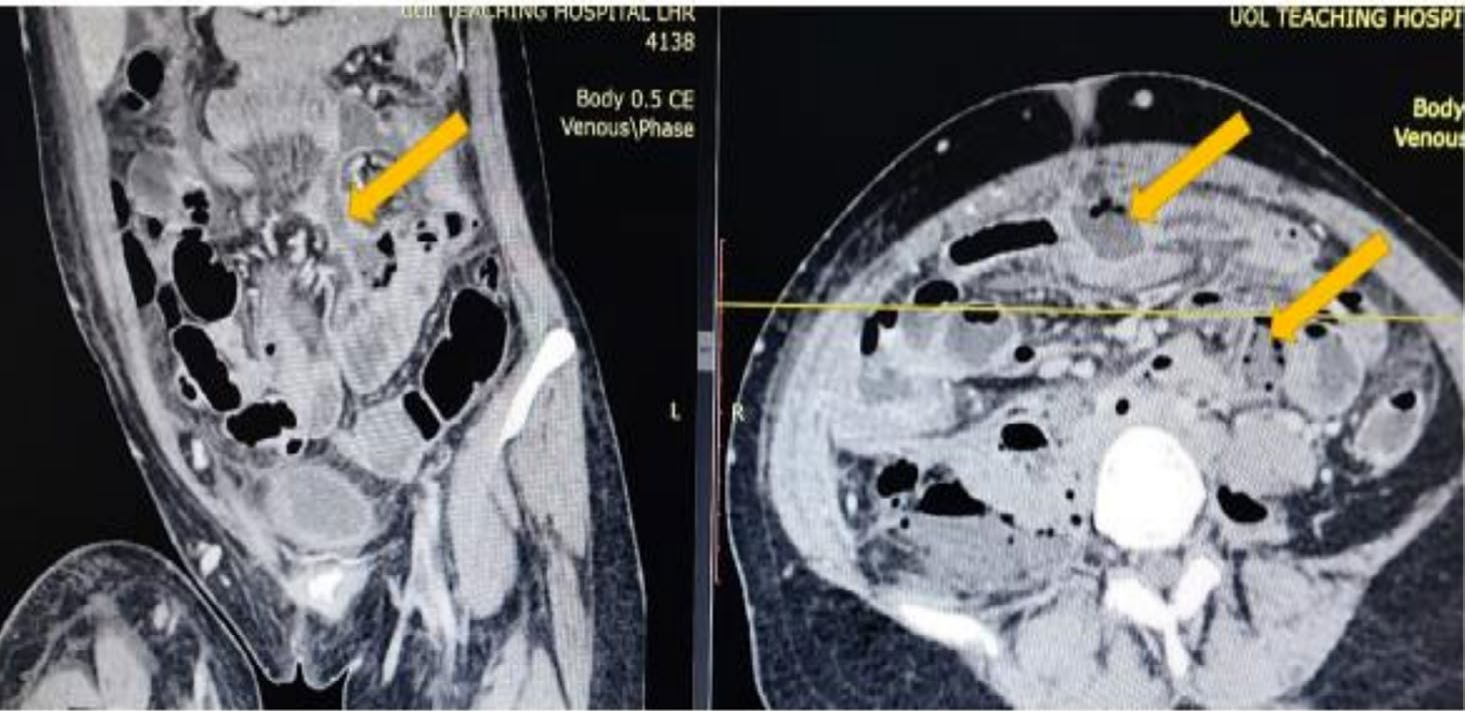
Communication between ileum and abscess cavity. An axial contrast‐enhanced CT image with oral contrast media. The image demonstrates a thickened and inflamed loop of the distal ileum immediately adjacent to the large right‐sided psoas abscess. A faint tract and inflammatory stranding can be seen between the bowel loop and the abscess cavity, confirming the site of the perforation and fistulous communication.
